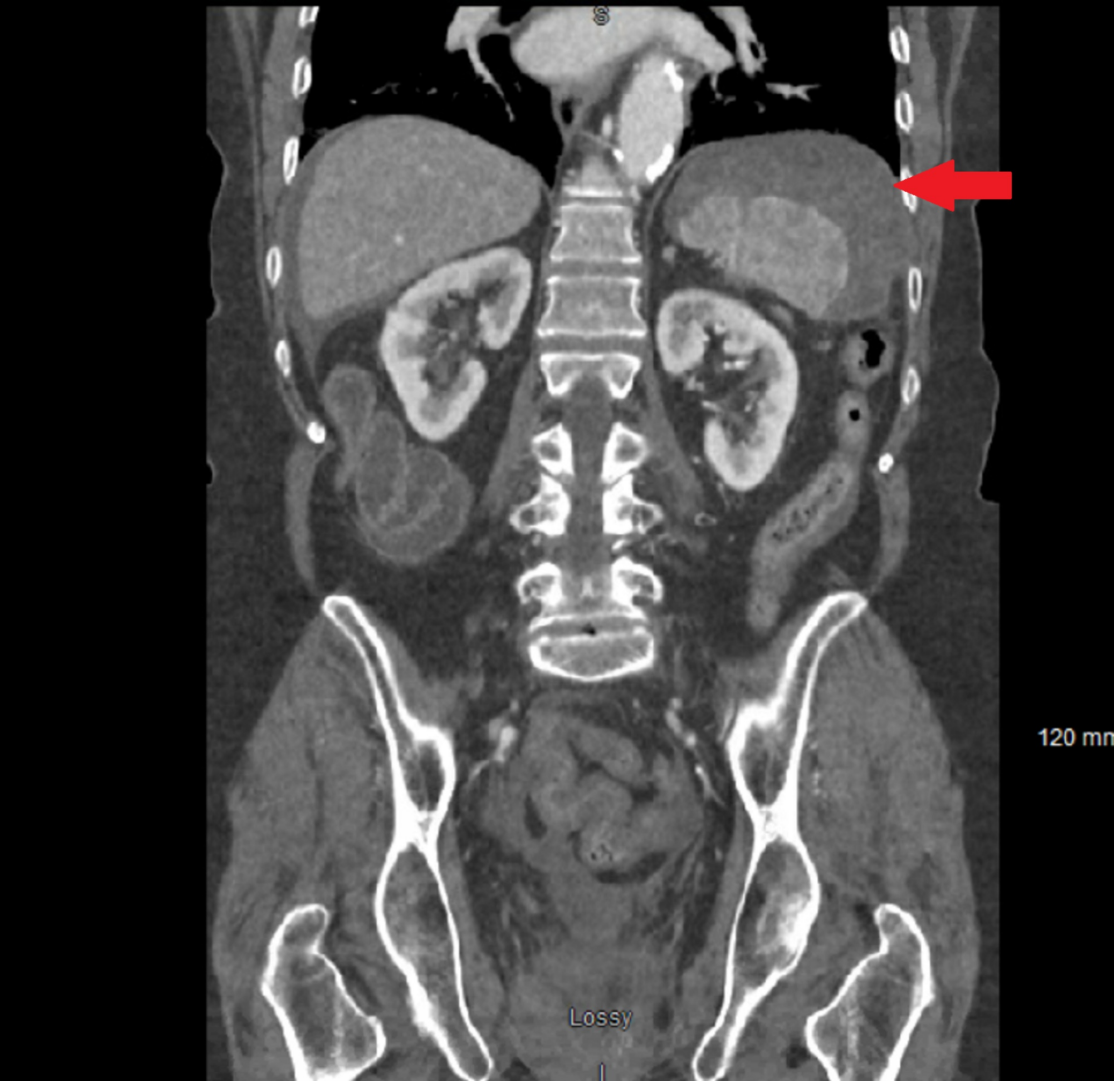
Contrast-enhanced abdominal computed tomography (coronal view) with a red arrow showing perisplenic fluid
